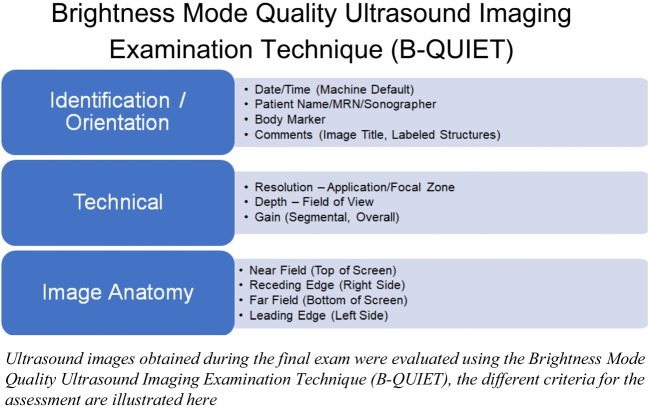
Evaluation criteria of the brightness mode quality ultrasound imaging examination technique (B-QUIET).
